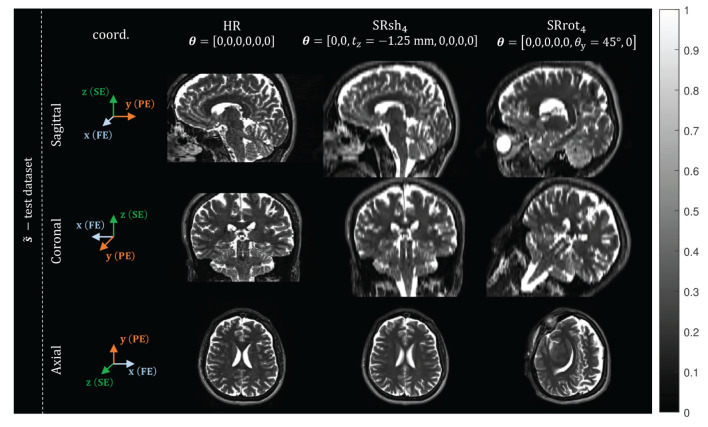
Examples of intensity-normalized acquired MS images: views from orthogonal planes of one of the MS images from the test dataset, for each protocol. The MR image coordinate system is reported in the first column, where FE, PE, and SE, represent the frequency-encoding (x-axis), phase-encoding (y-axis), and slice-encoding (z-axis) directions, respectively. For each MS image, the corresponding rigid transformation parameter vector θ is shown.
